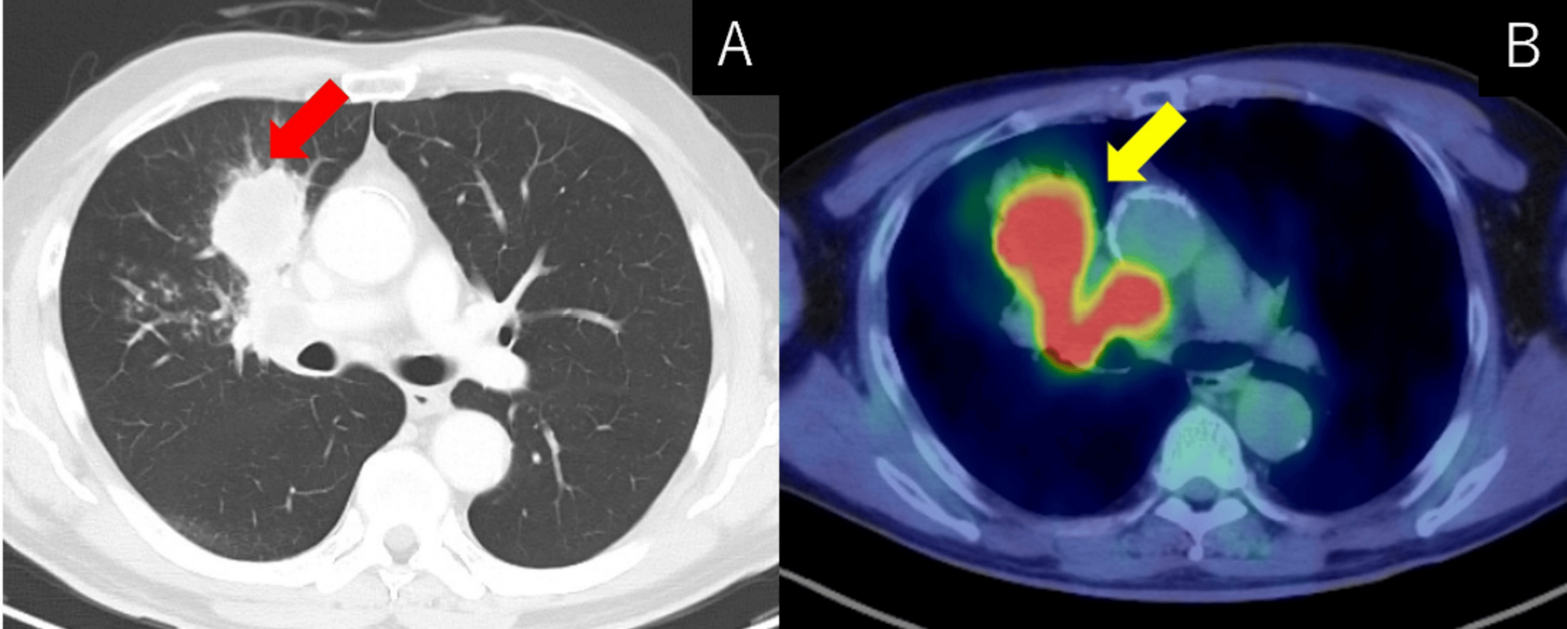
Radiological finding before treatment (A) Computed tomography (CT) revealed a mass in the right middle lobe and enlarged mediastinum lymph node (red arrow). (B) Positron-emission tomography (PET)-CT revealed increased uptake in the right middle lobe and mediastinum lymph node (yellow arrow).
